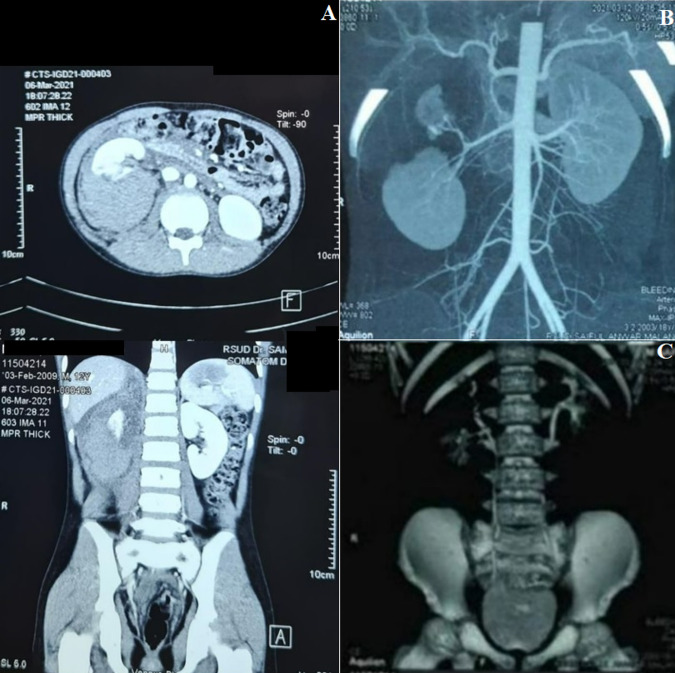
evaluation and clinical findings in the first patient A) initial CT scan showed viable upper, middle, and lower renal pole with intact contralateral kidney: coronal view (upper), axial view (lower); B, C) CT scan evaluation, intact vascular pedicle (upper), intact pelvicalyceal system (lower)
